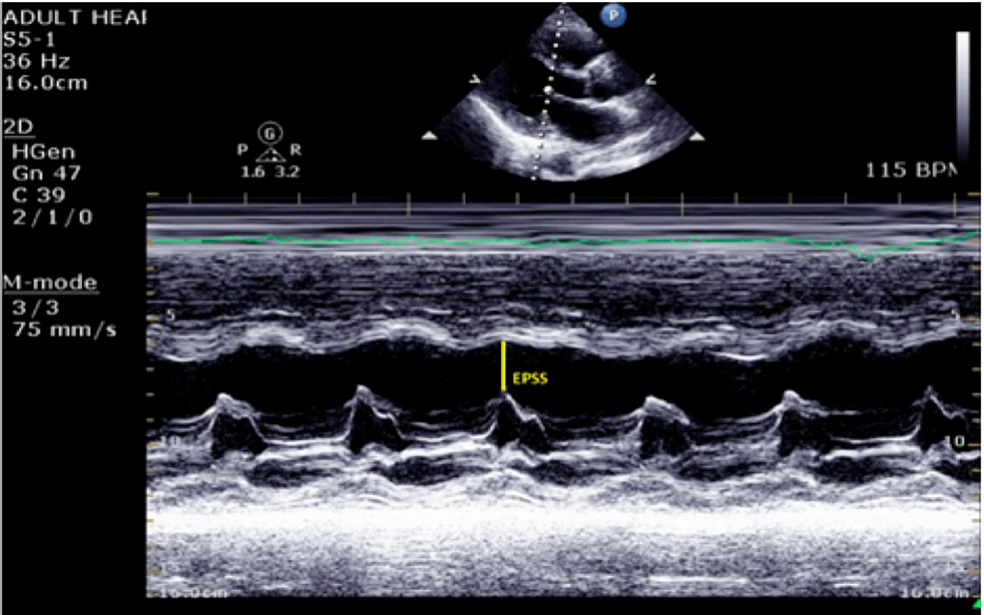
M-Mode on echocardiography denoting left ventricle dysfunction as evidenced by increased EPSS. EPSS: E-point septal separation
